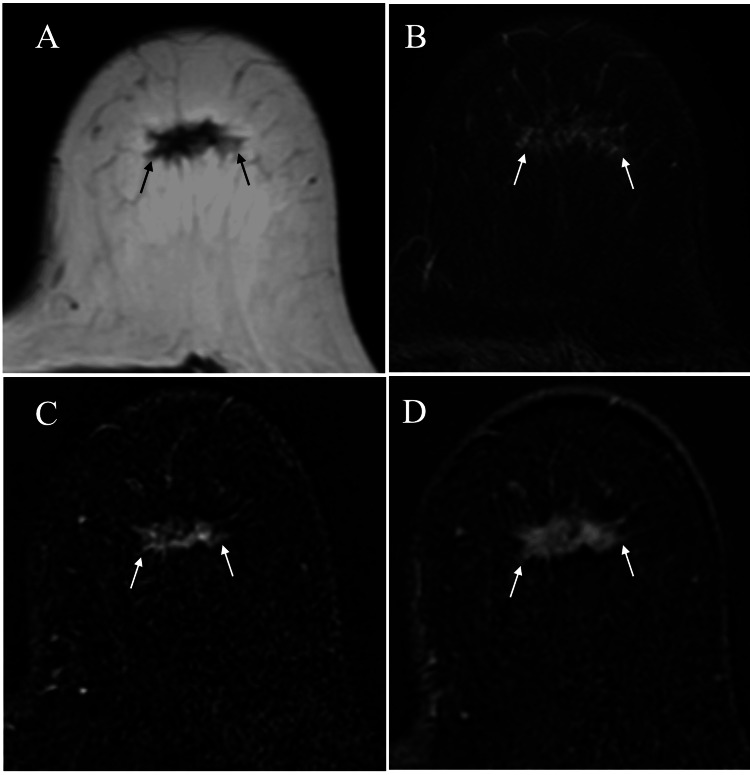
MRI findings MRI of the mass showed low signals (arrows) on T1-weighted images (A), faint high signals (arrows) on T2-weighted images (B), and early (C) and retained (D) enhancement (arrows) on dynamic studies MRI: magnetic resonance imaging
